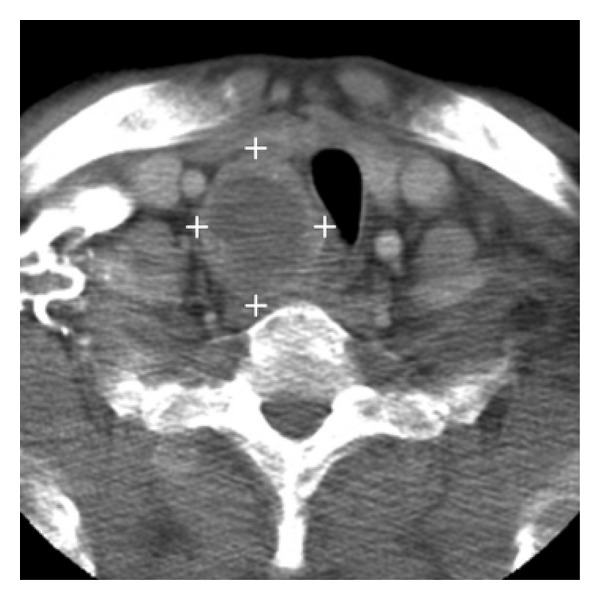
Computed tomography finding of parathyroid carcinoma (transverse)—a cystic tumor in the upper mediastinum, tracheal deviation.
